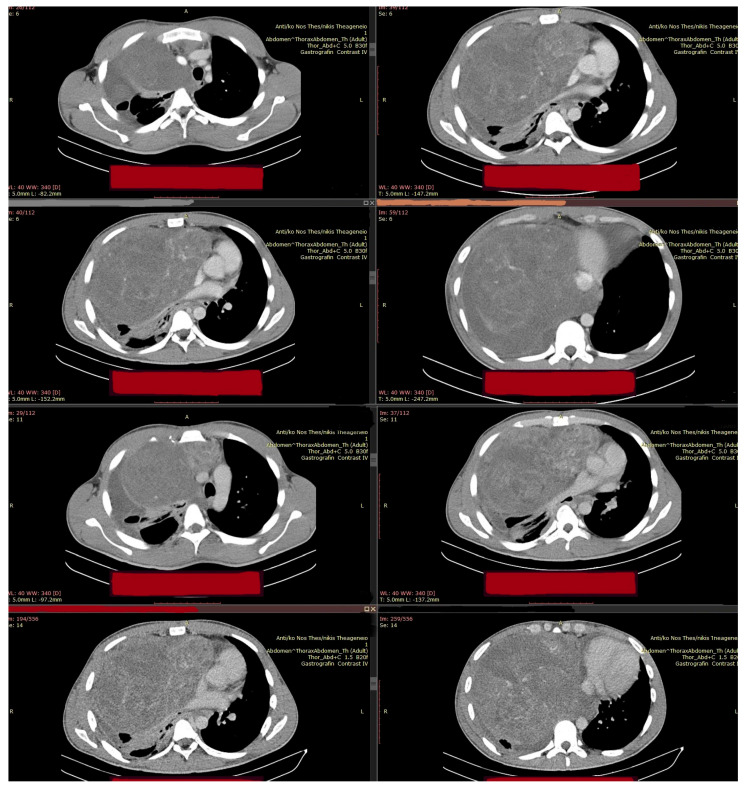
Preoperative (pro median sternotomy, extended with complementary “T-Shaped” mini anterior (R) thoracotomy) Chest-CT.
